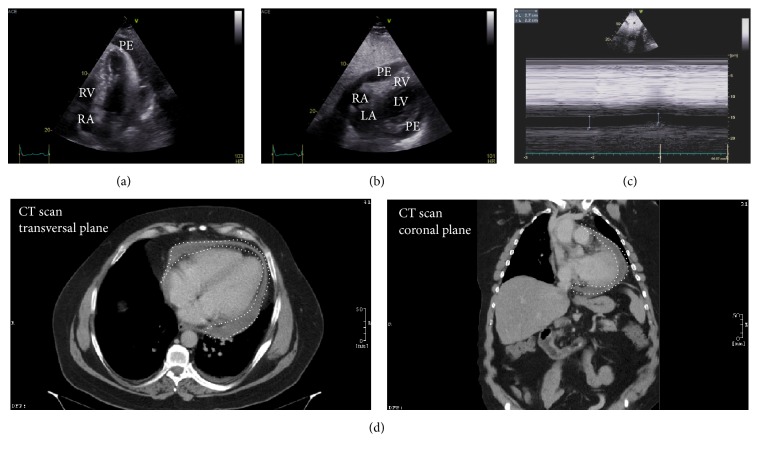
TTE demonstrating intermediate pericardial effusion leading to pericardial tamponade. (a) Apical four-chamber view showing intrapericardial pressure exceeding RA systolic pressure as evidenced by systolic RA collapse. (b) Subcostal view demonstrating diastolic RV collapse. (c) M-mode echocardiography of dilated inferior vena cava (27 mm) with <50% inspiratory reduction. (d) CT with contrast agent reveals residual circumferential pericardial effusion after pericardiocentesis. Pericardial effusion is highlighted by a dotted white line. Structures: pericardial effusion (PE), right atrium (RA), right ventricle (RV), left atrium (LA), and left ventricle (LV).
